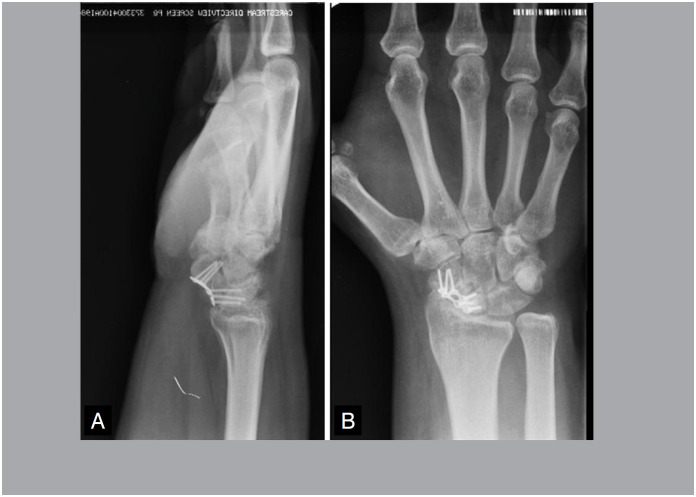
Postoperative pseudarthrosis fixation X-ray of the scapula.
